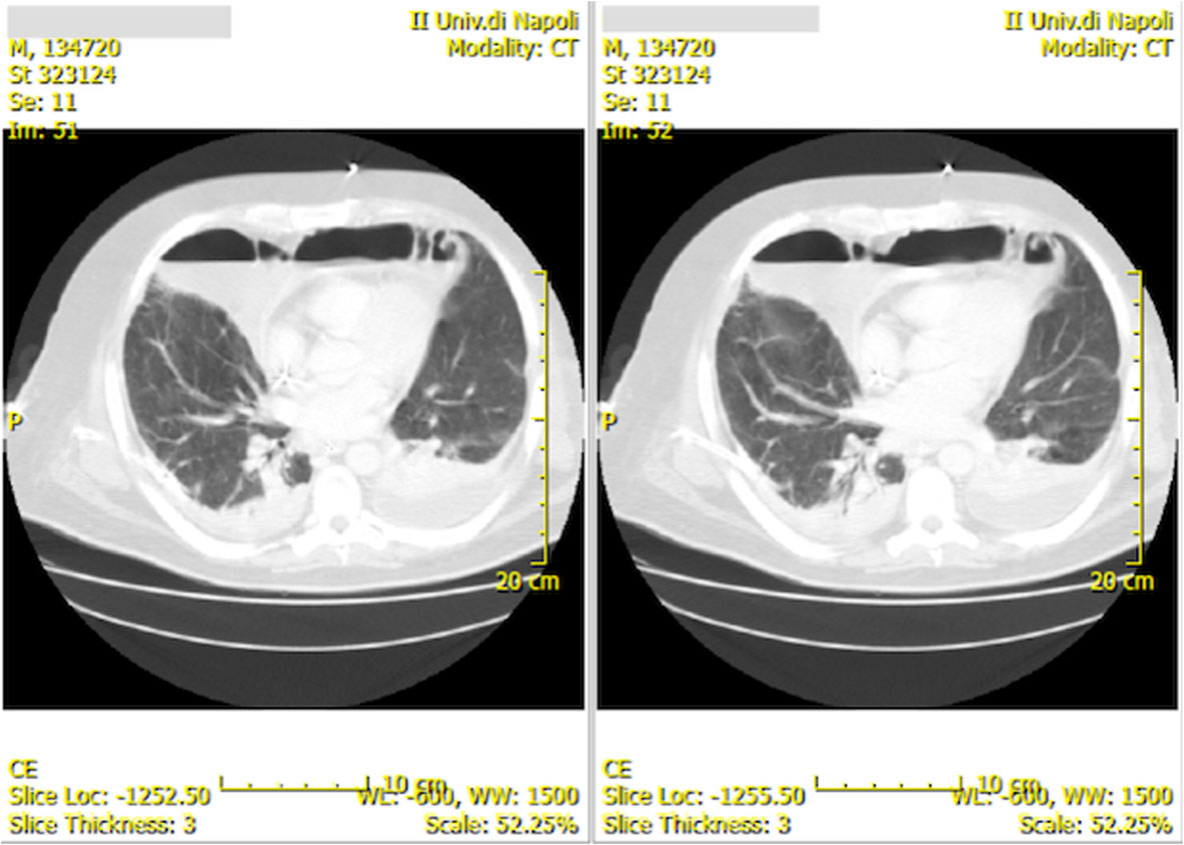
Cervical computed tomography scan
